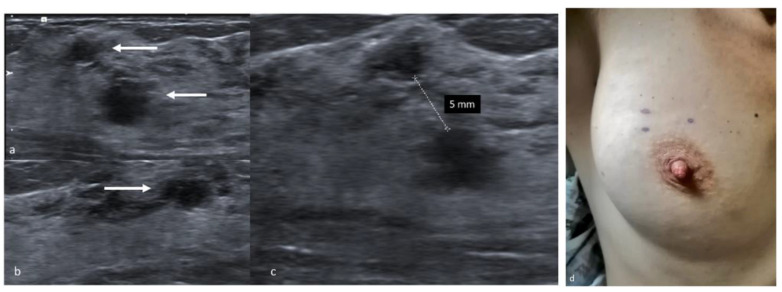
Preoperative skin tattoo. Transverse sonogram showing hypoechoic, round shaped multifocal masses with indistinct margins in the upper outer quadrant of the right breast (a,b, arrows). The distance between separate lesions is measured (c). The dermographic skin markers of the tumor’s projection on the skin surface (d).
